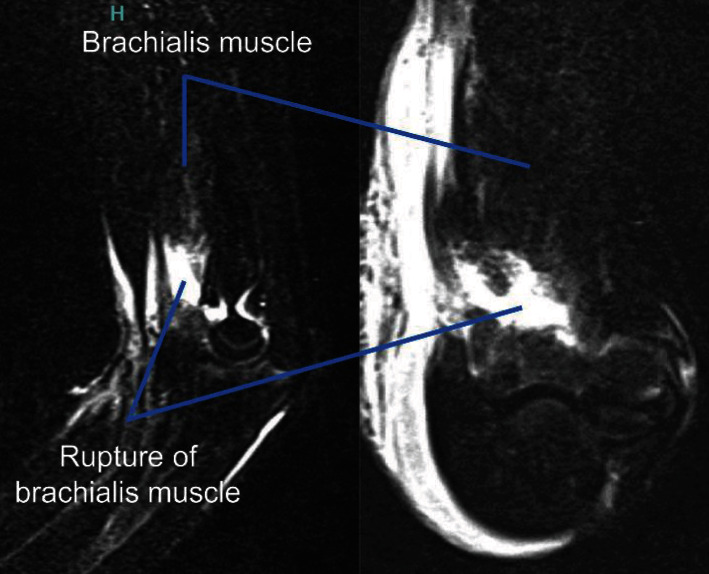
Short-TI inversion recovery magnetic resonance imaging. Brachialis muscle was ruptured completely.
